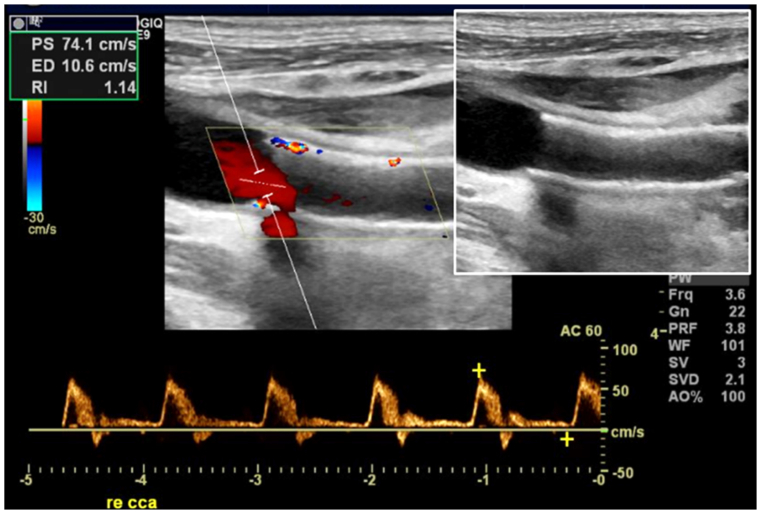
Postoperative duplex ultrasound image (small window: without color).
